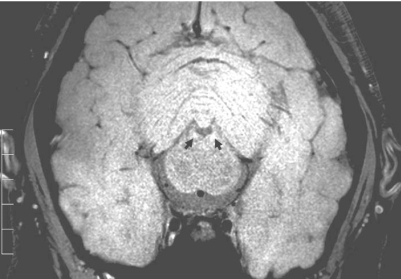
Magnetic resonance image showing a cross-section of the upper pons displaying the loci coerulei (LC). A picture was taken in a healthy human subject with the modification of the method of Sasaki *et al*., 2006, on a 3-tesla scanner to obtain neuromelanin signal to identify LC. The loci coerulei are shown by the small white areas in the bottom corners of the fourth cerebral ventricle, indicated by black arrows. By courtesy of Professor D. Auer, Queen’s Medical Centre, Nottingham.
